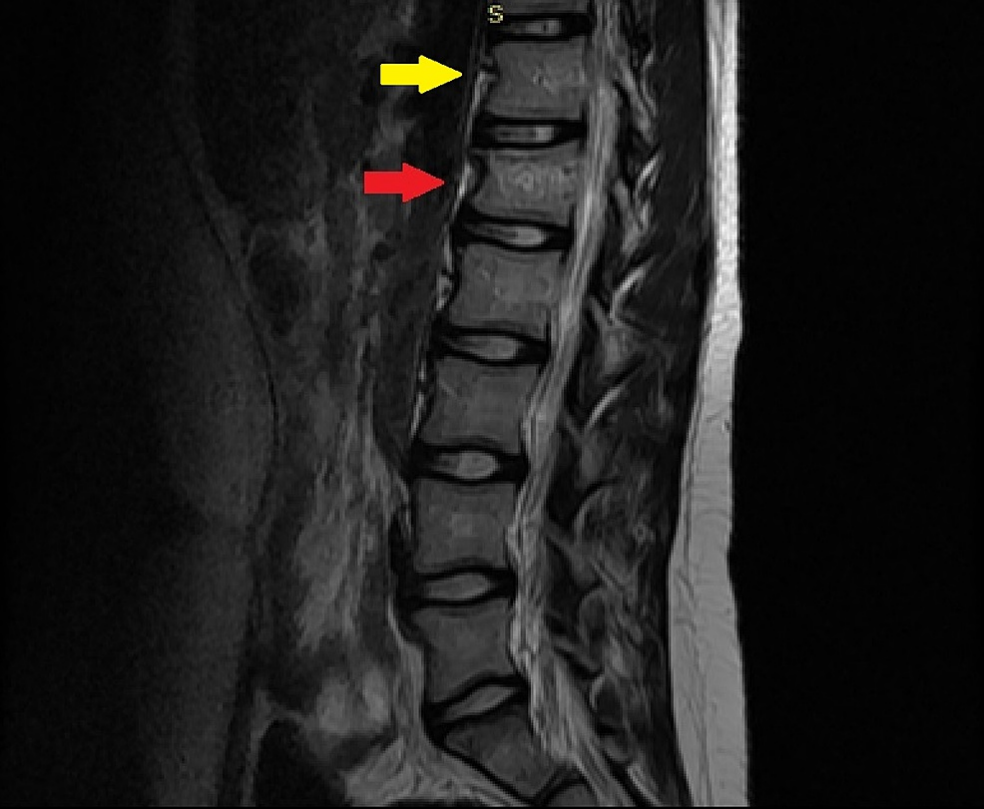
T2 sagittal MR image of the lumbar spine. Wedge fracture of the T12 (yellow arrow) and L1 vertebra with associated diffuse marrow edema (red arrow).
